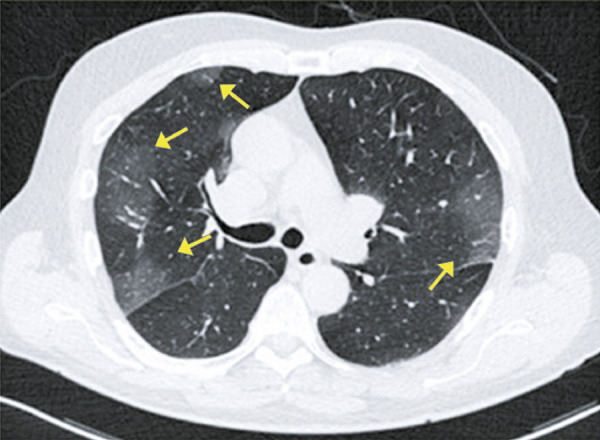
A 75-year-old patient with COVID-19, presenting respiratory discomfort for 3 days, and fever for one day. Computed tomography showing peripheral ground-glass opacities in the upper lobes
